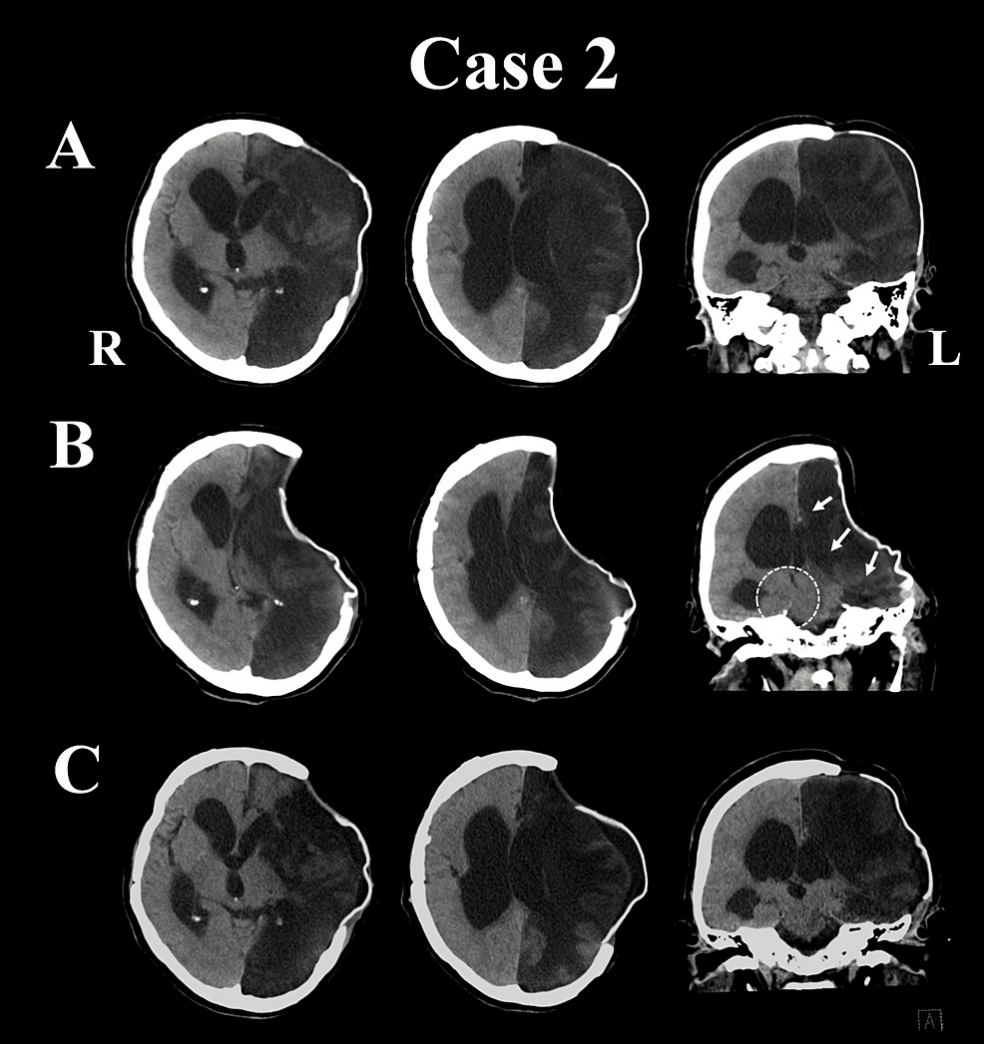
CT findings in Case 2 (A) There is a significant enlargement of the lateral ventricles, especially on the right. The skin at the site of the decompressive craniectomy is bulging as a result of the diffuse edema of the left hemisphere. (B) The skin at the site of the bone defect has sunk considerably. The midline shift contralateral to the bone defect seems mild; however, because of the inward and downward pressure at the site of the bone defect (white arrows), the right side of the midbrain is compressed by the cerebellar tent (white circle). (C) The size of the right ventricle is mildly decreased and that of the left ventricle is enlarged. There is improvement in the midline shift and compression of the midbrain by the cerebellar tent. CT: computed tomography
